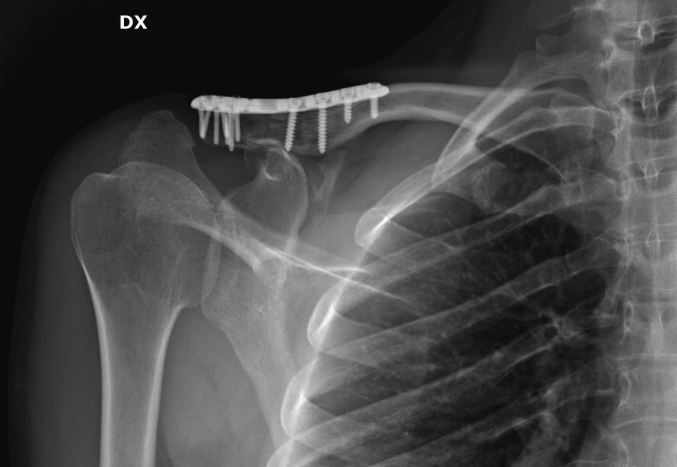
Xray clavice after surgery.
